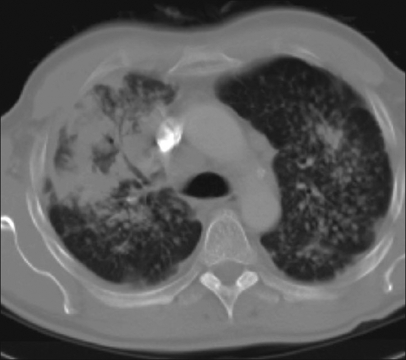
Contrast-enhanced CT (Lung window settings)What is the differential diagnosis?What further investigations would you perform to confirm the diagnosis?
